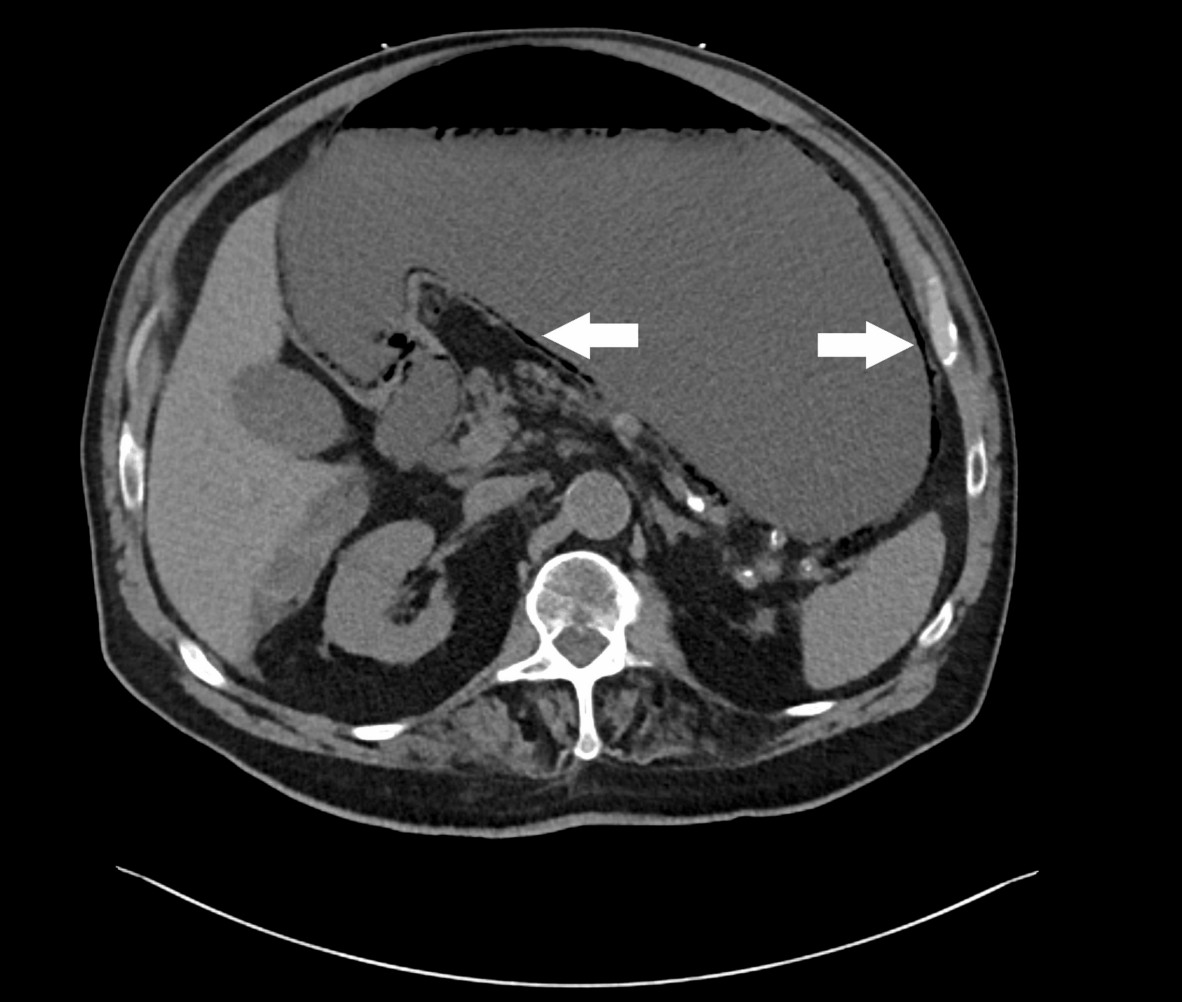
CT abdomen demonstrating gastric pneumatosis Hypodense air in the wall of the stomach is highlighted by the arrows.
